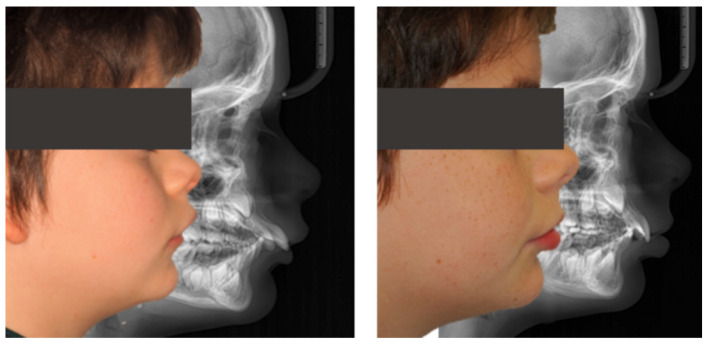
Initial and final lateral photographs and cephalometric radiographs of a 9-year-old male patient treated with Invisalign^®^ First.
